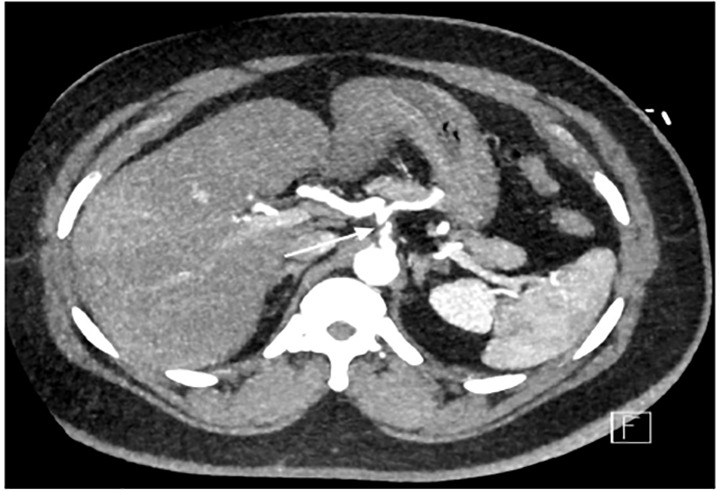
An axial image of a contrast-enhanced computed tomography angiogram showing a dissection flap in the celiac trunk (arrow).
